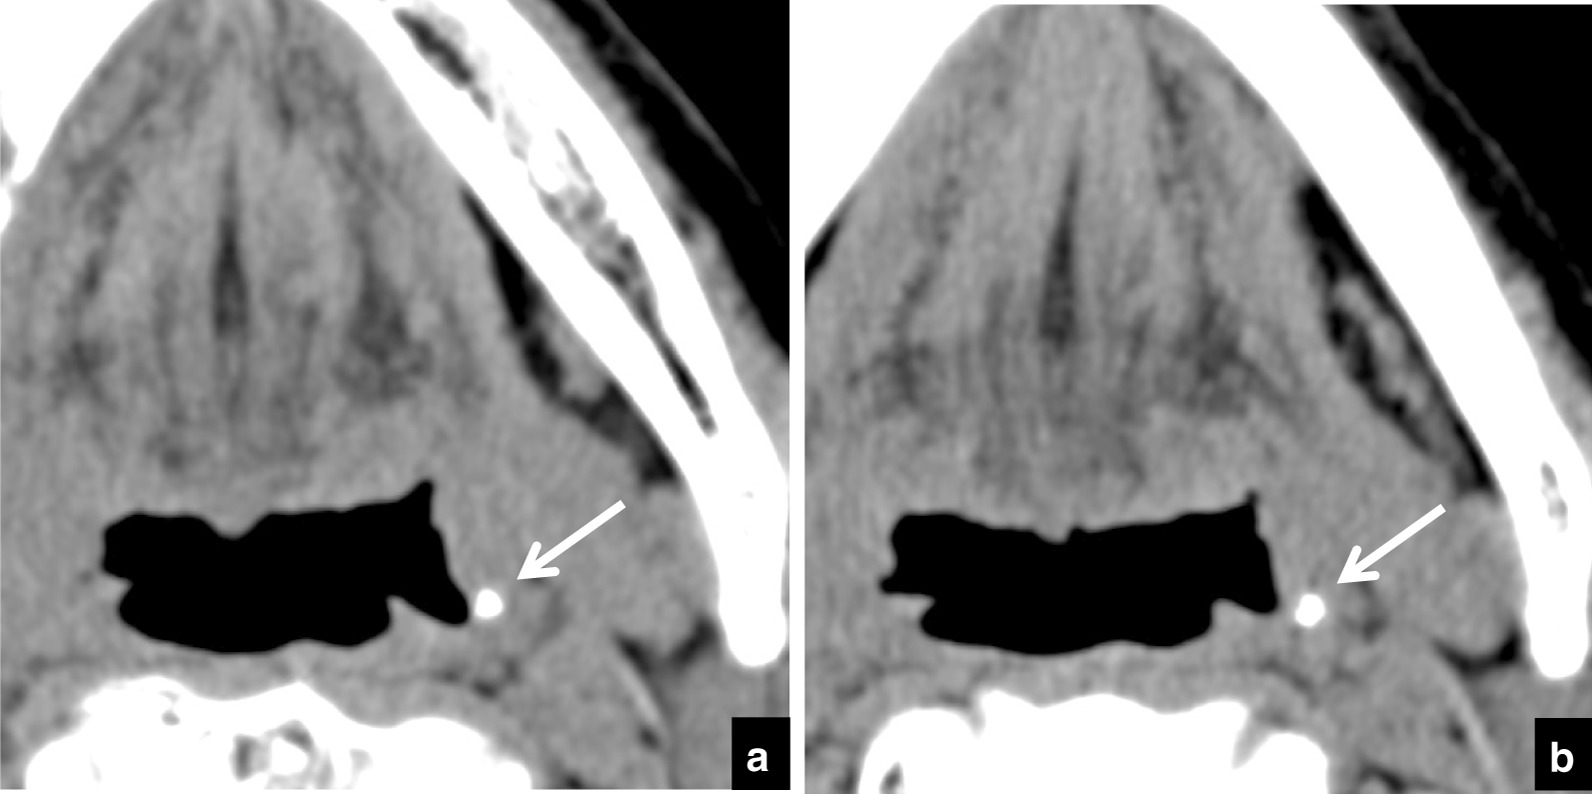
An 80-year-old subject with malignant tumor of the right mandible in whom dynamic movement of the tonsillolith was seen toward the external side on CT. a Axial CT image at the palatine tonsillar level at the initial examination. The depth of the tonsillolith (arrow) is 0.7 mm. b Follow up CT image at the same level 6 months later. The depth of the tonsillolith (arrow) is now 1.8 mm, following movement in the lateral direction (away from the respiratory tract)
